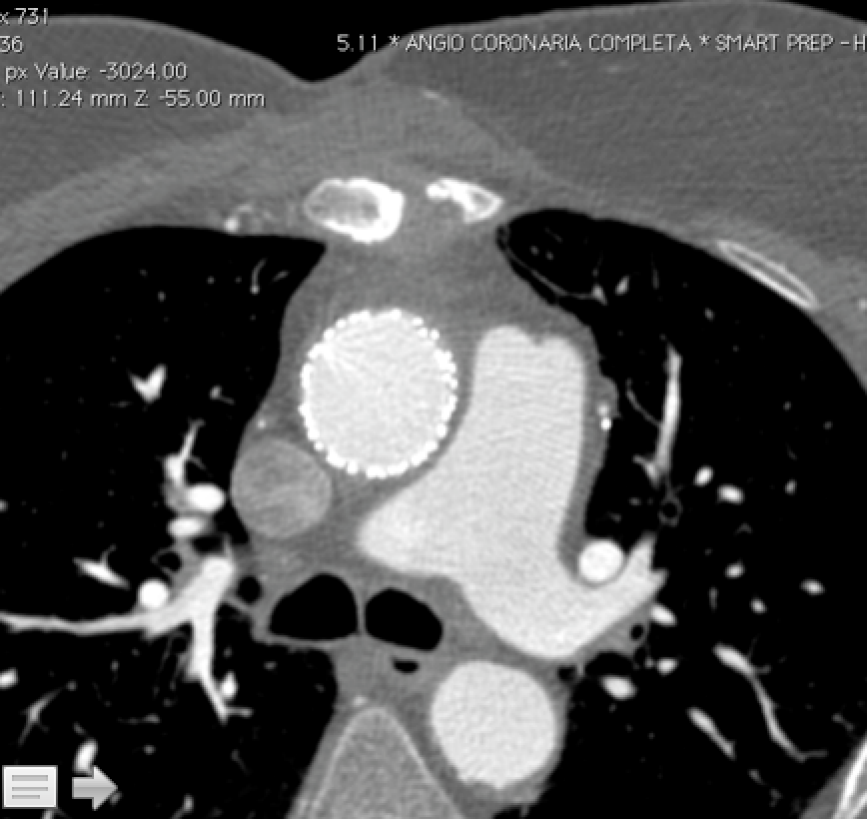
Computed tomography, axial view, post stent graft in ascending aorta.
